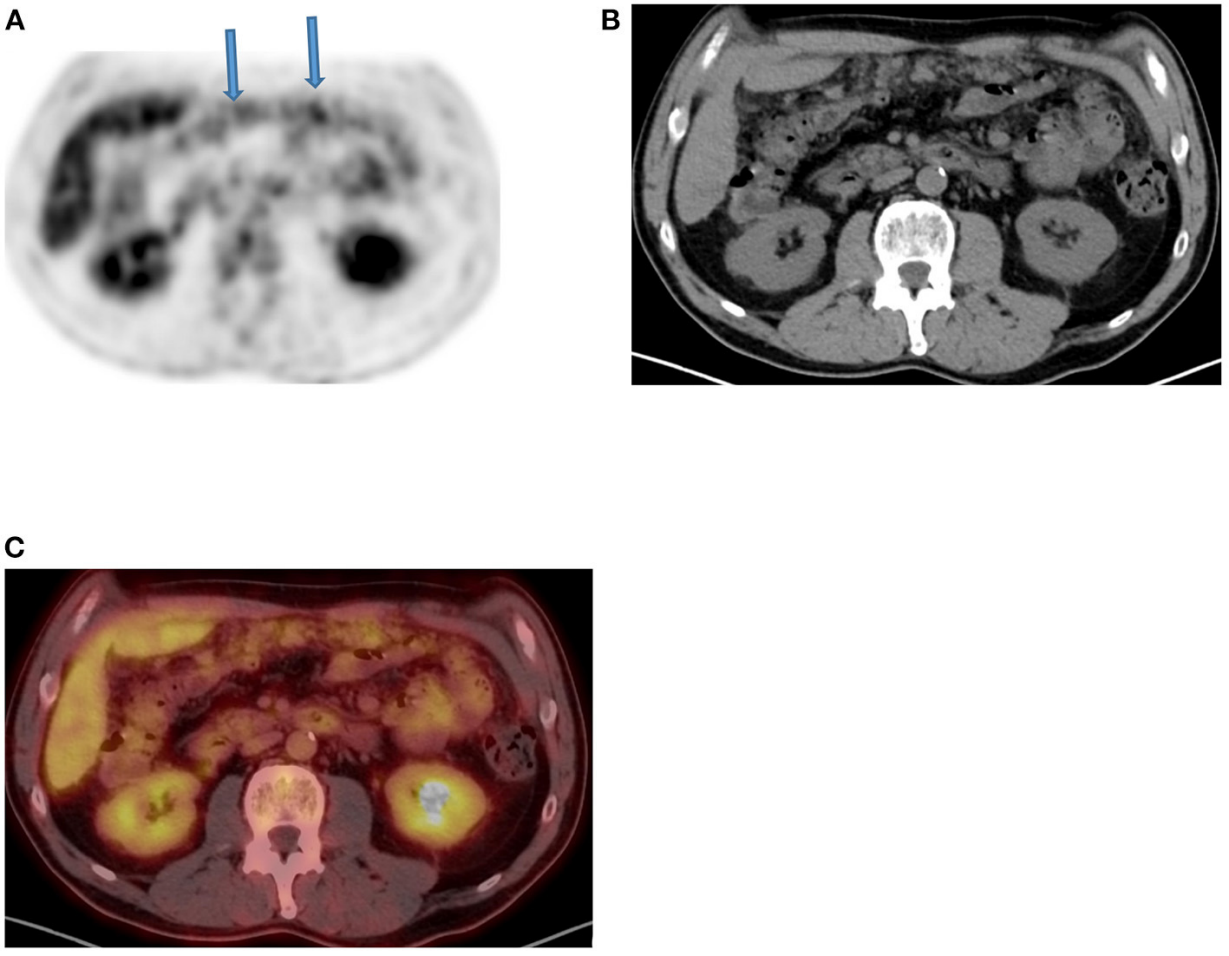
Omental cake. FDG PET Axial (A) image demonstrates diffuse moderate increased linear uptake in anterior abdomen in a “band like” patterns (arrows) that mimic large bowel uptake, in a patient with ovarian carcinoma who was referred for therapy response evaluation. Corresponding CT (B) and combined PET/CT (C) confirmed the features of “omental cake” that are suggestive of peritoneal metastasis.
